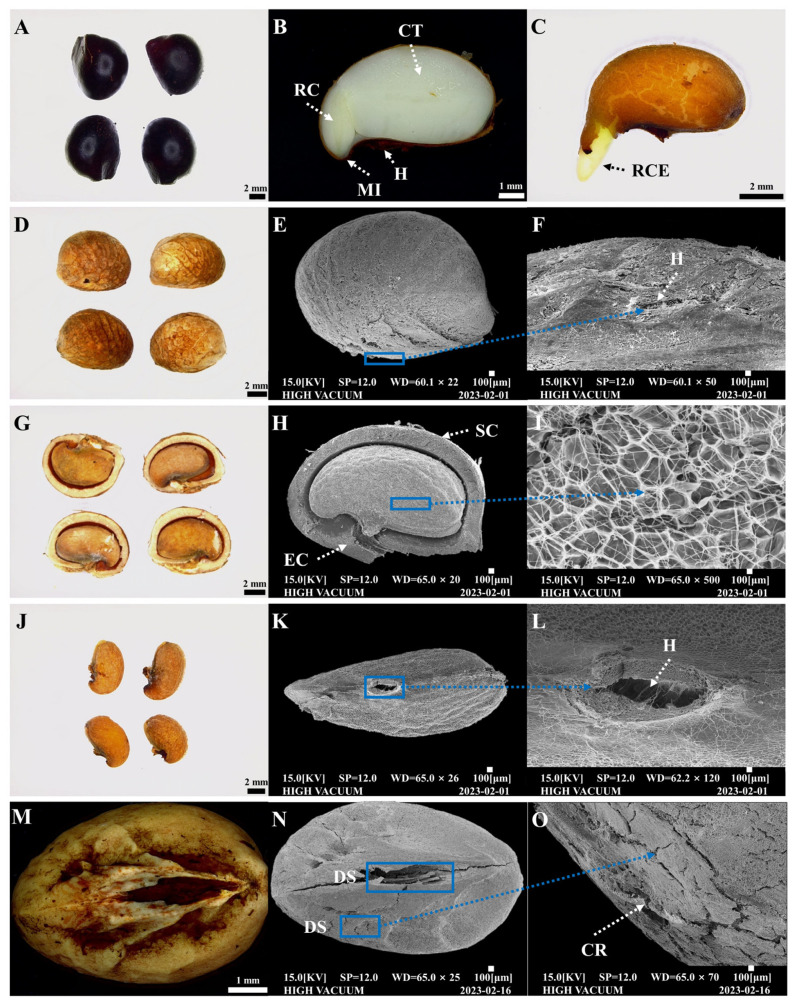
Morphology and anatomy of *Rhodotypos scandens* (Thunb.) Makino seeds. Seed with pericarp (A), internal view (B), radicle emergence (C), seed without pericarp (D–F), seed without endocarp (G–L), and seed scarified with sulfuric acid (M–O). CR, cracks; CT, cotyledon; DS, damaged surface; EC, endocarp; H, hilum; MI, micropyle; RC, radicle; RCE, radicle emergence; SC, seed coat.
